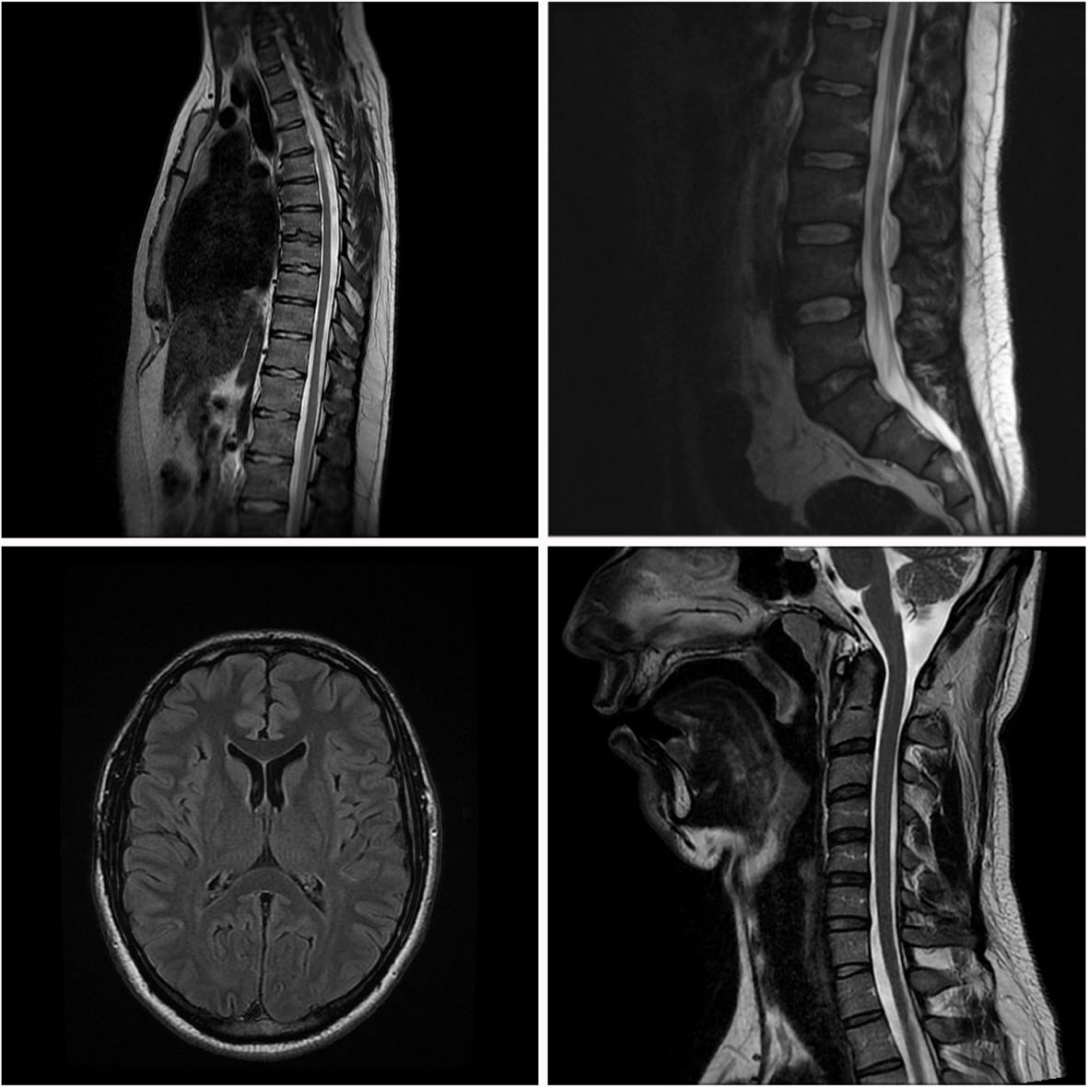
Magnetic resonance imaging scan of the brain and cervical, thoracic and lumbar spine (SIGNA Voyager 1.5T)
